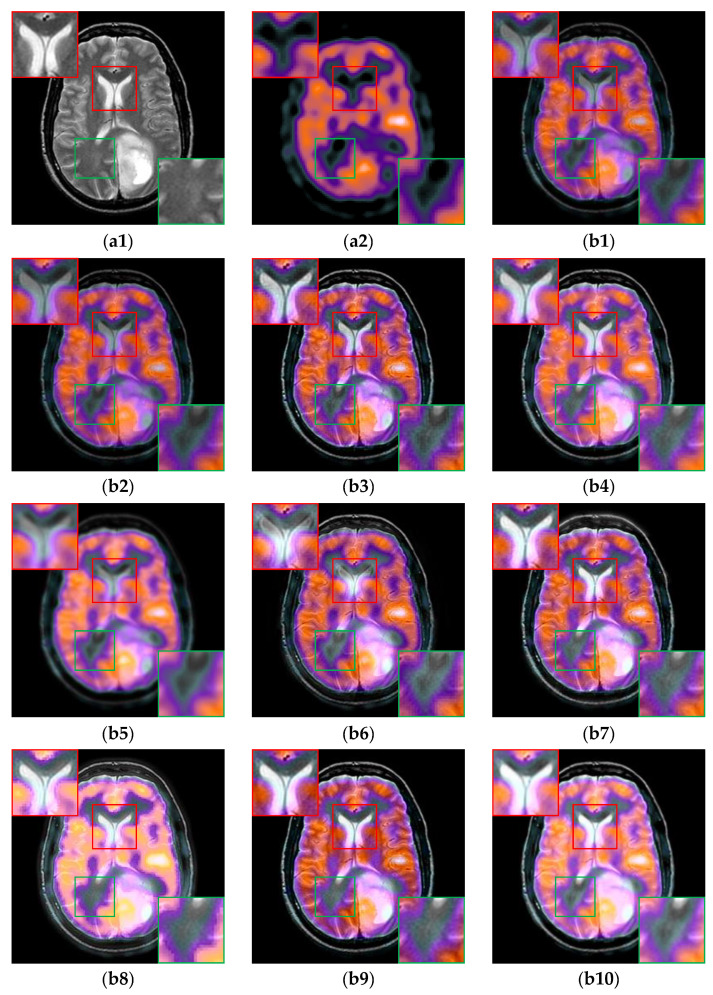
The results of all algorithms on the SPECT-CT/MR image pairs from Atlas dataset. (a1) Source CT/MR image; (a2) Source SPECT image; (b1) U2Fusion; (b2) DenseFuse; (b3) IFCNN; (b4) NestFuse; (b5) RFN-Nest; (b6) PAPCNN; (b7) ReLP; (b8) TIF; (b9) CDDFuse; (b10) CIRF.
